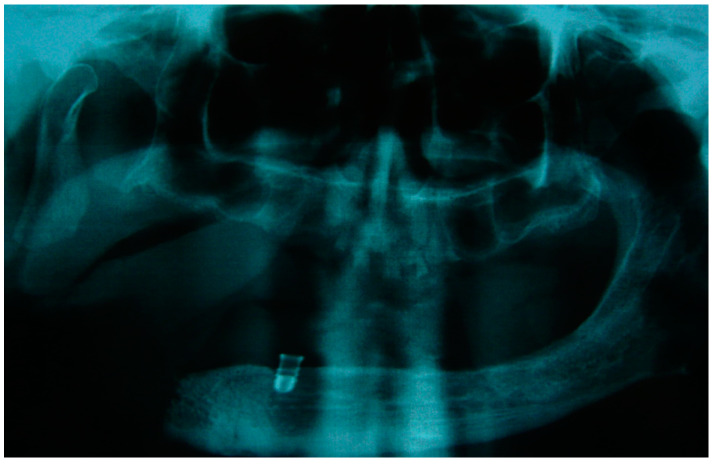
Patient with hemimandibulectomy L1,2,3 in orthopanoramic X-ray.
